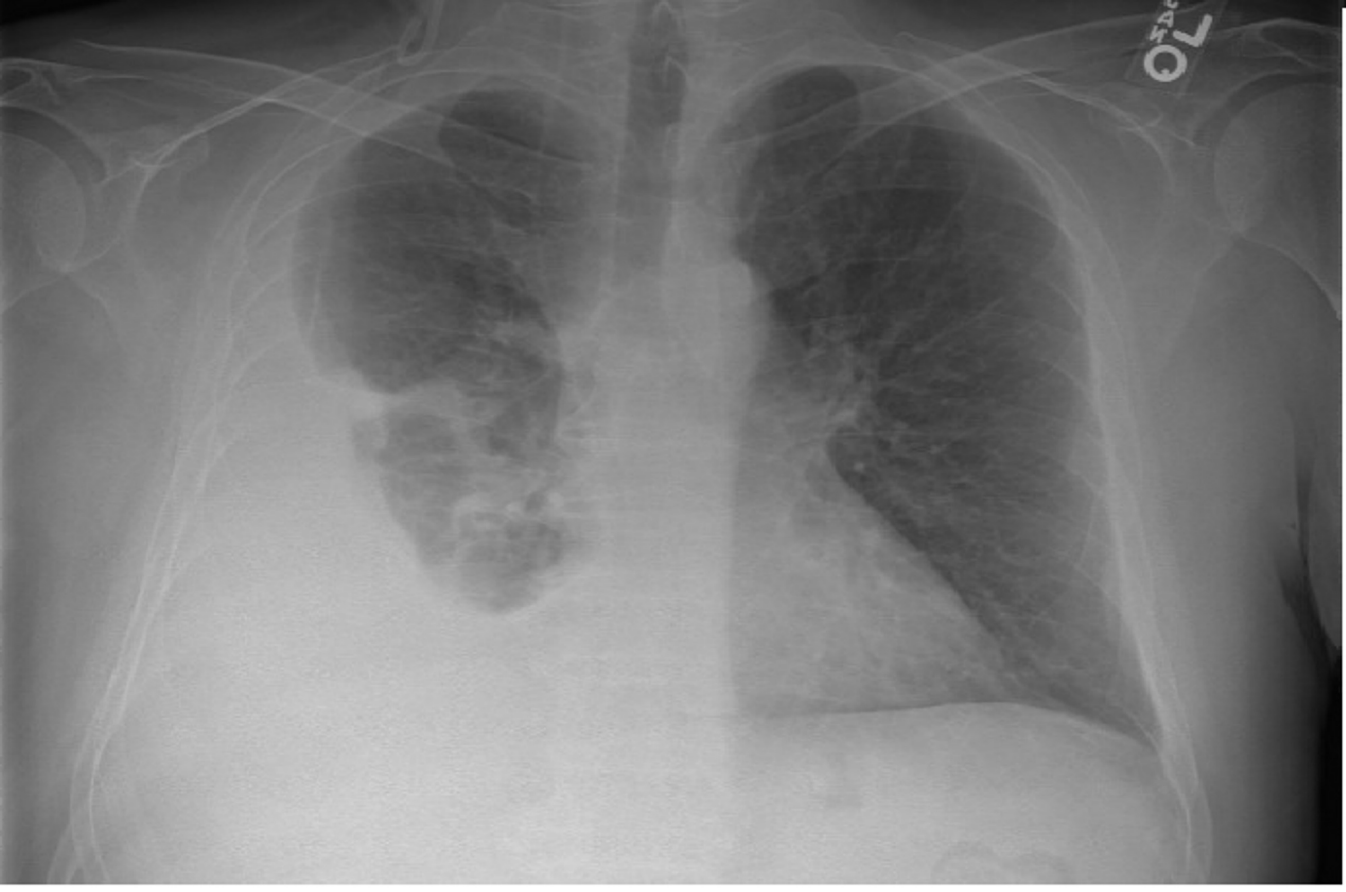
Chest X-ray showing a moderate-sized right-sided pleural effusion
